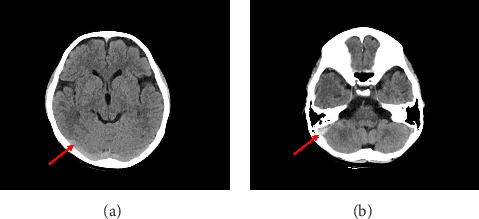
The CT images of patients after onset (a, b). Axial CT images 60 h after onset, showing increased attenuation intensity in the right transverse sinus and sigmoid sinus (red arrows), with no significant brain parenchymal abnormalities.
